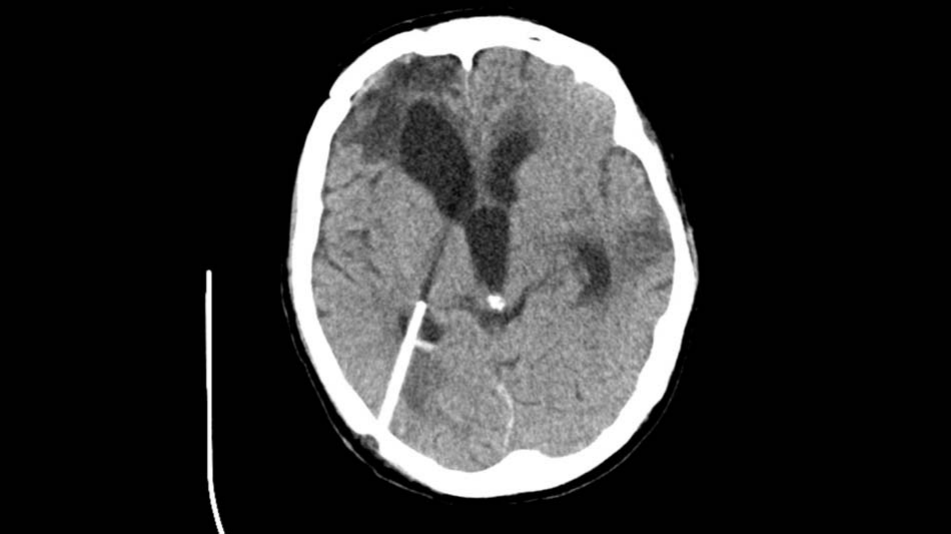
Head CT—state after VP shunt implantation. (Department of Neurosurgery own material).
